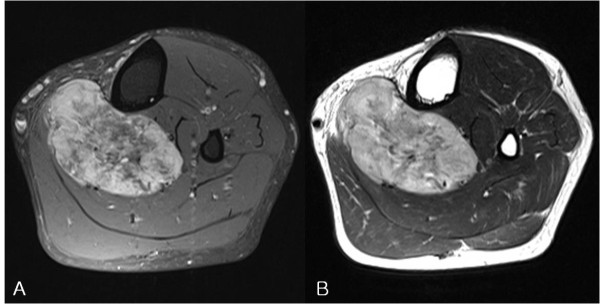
MRI images of an alveolar soft part sarcoma. (A) An axial T1-weighted fat-suppressed image and (B) an axial T2-weighted image. High signal on T1FS, T2WI with multiple signal voids are apparent.
